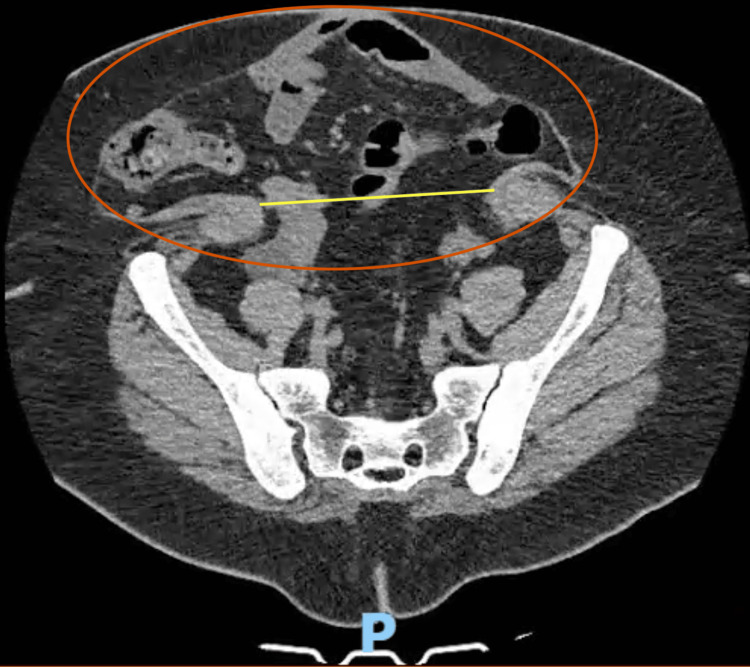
CT scan showing hernia sac measuring 28 x 11 x 25 cm (red circle), with a lateral-to-lateral wall defect measuring 16 x 18 cm (yellow line) CT: computed tomography
